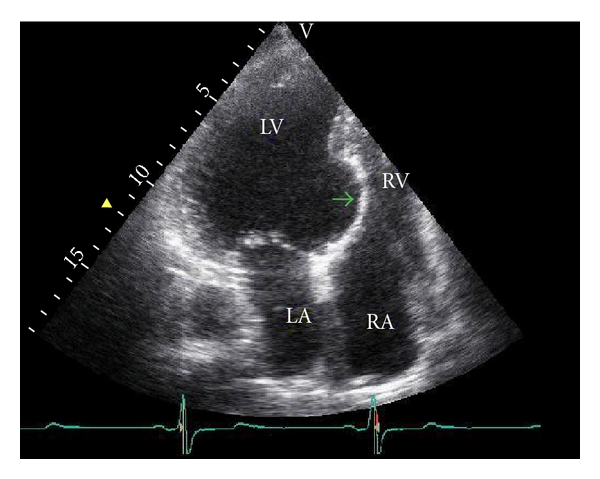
Transthoracic echocardiographic image of left ventricular septal aneurysm. LA: left atrium, RA: right atrium, LV: left ventricle, RV: right ventricle.
